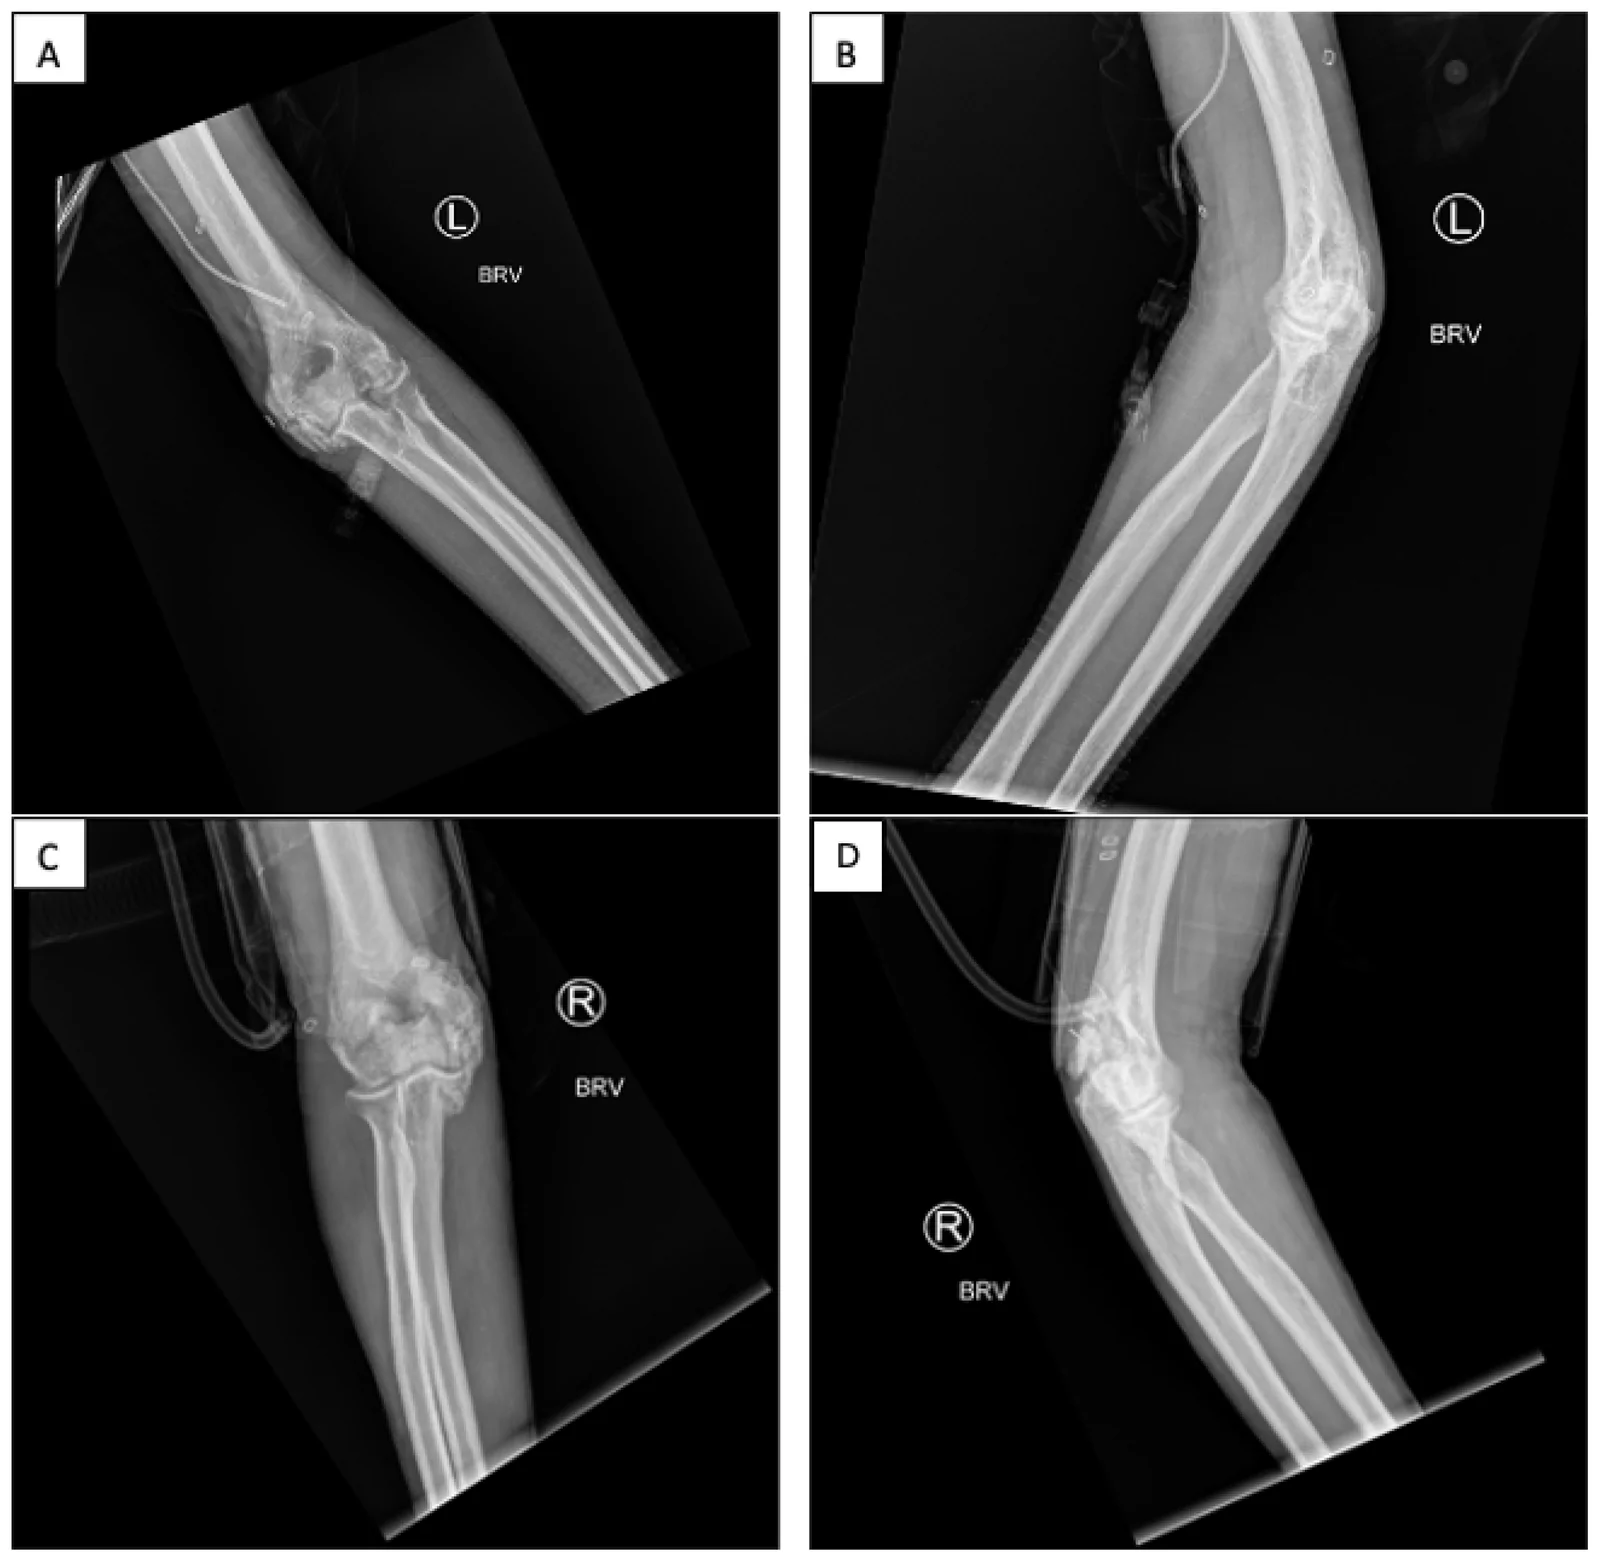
Preoperative (A,C) anterior to posterior (AP) radiographs of the left and right upper extremities, respectively. (B,D) Lateral radiographs of the left and right upper extremities, respectively. This figure demonstrates severe, bridging heterotopic ossification about the ulnohumeral joint bilaterally upon initial presentation.
